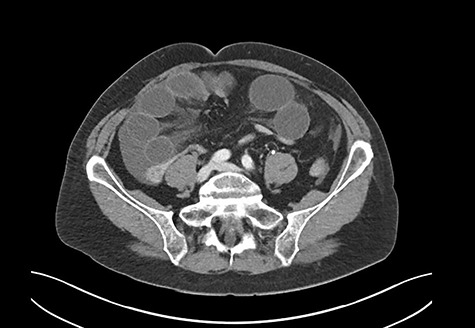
CT scan: severe mechanical obstruction of the small intestine caused probably by adhesions.
